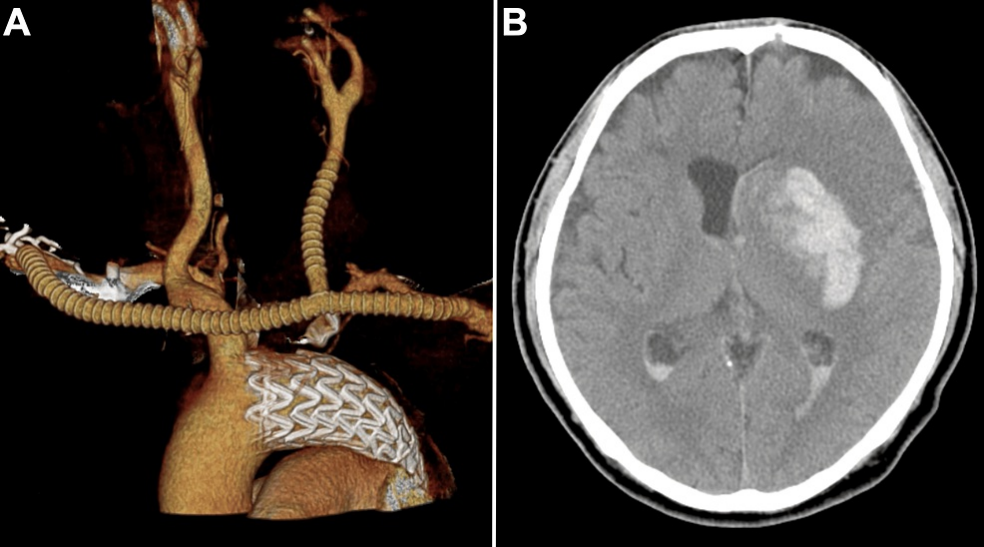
Postoperative computed tomography and computed tomography angiography. Ligation of the proximal part of the left common carotid artery (CCA) and bypass from the right axillary to the left axillary artery to the distal part of the left CCA (A). Postoperative computed tomography showed hemorrhage in the left putamen and caudate nucleus (B).
